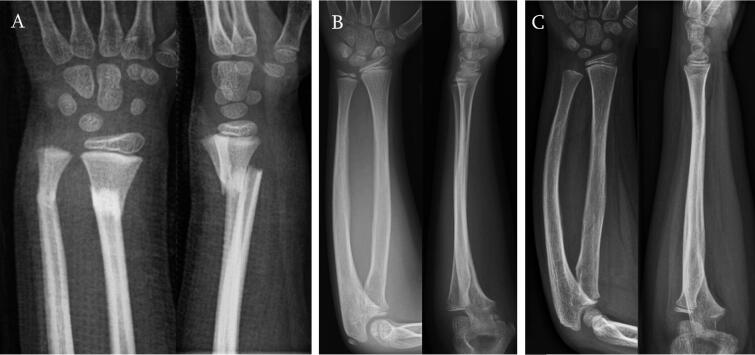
A–C: all 3 patients’ fractures were immobilized in bayonet position (A) without reduction with synthetic dorsal above-elbow and volar below-elbow splints applied in finger-trap traction without anesthesia in the emergency department. Splints were removed at 4 weeks. Radiographs at 3.5 years from the injury in case 1 (B) and case 3 (C). Parents of Case 2 (A) did not want any follow-up radiographs taken, because their son had made full functional recovery and he had no pain.
